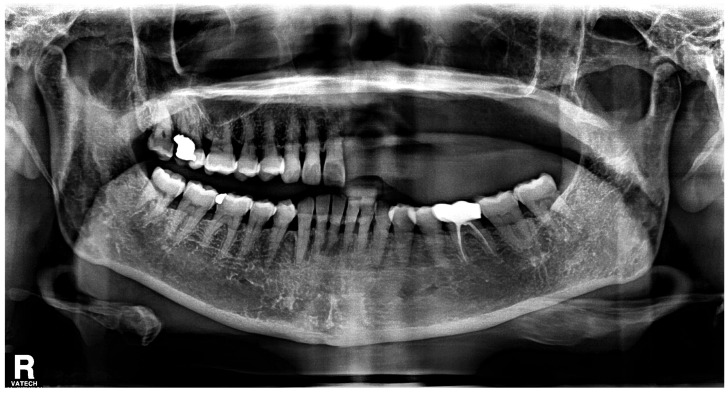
3 months post-operative panoramic radiograph shows no definitive abnormal finding.
